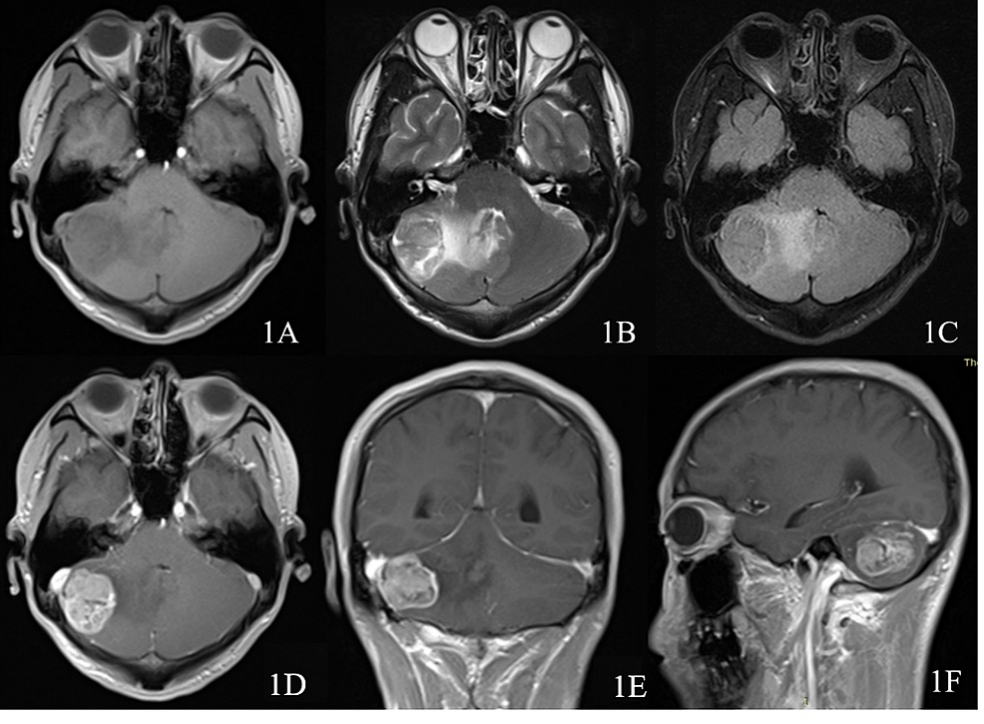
Preoperative examination Magnetic resonance imaging (MRI) scan showing tumor that is hypointense on T1WI, mixed hyperintense on T2WI, and iso-signal on fluid-attenuated inversion-recovery (1A-1C). Postgadolinium scan showing a significant but heterogeneous enhancement mass 35.4 mm × 30.5 mm × 30.3 mm (1D-1F).
